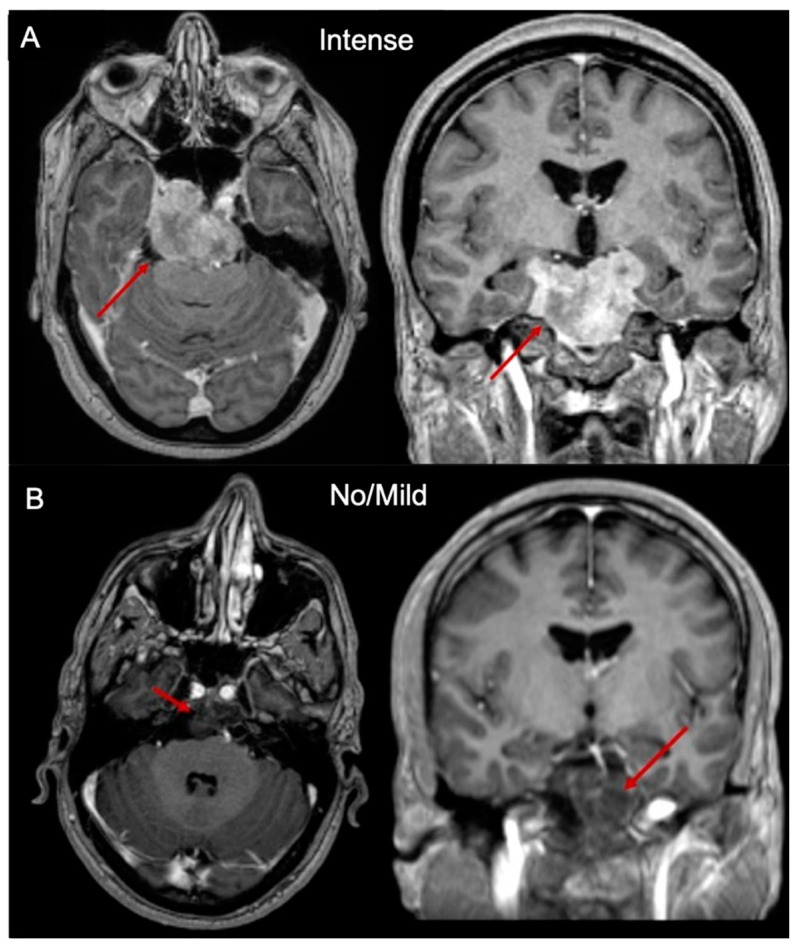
Axial and coronal T1-weighted post-contrast MR images showing SBC (red arrows) with intense (A) and no/mild (B) contrast enhancement.
